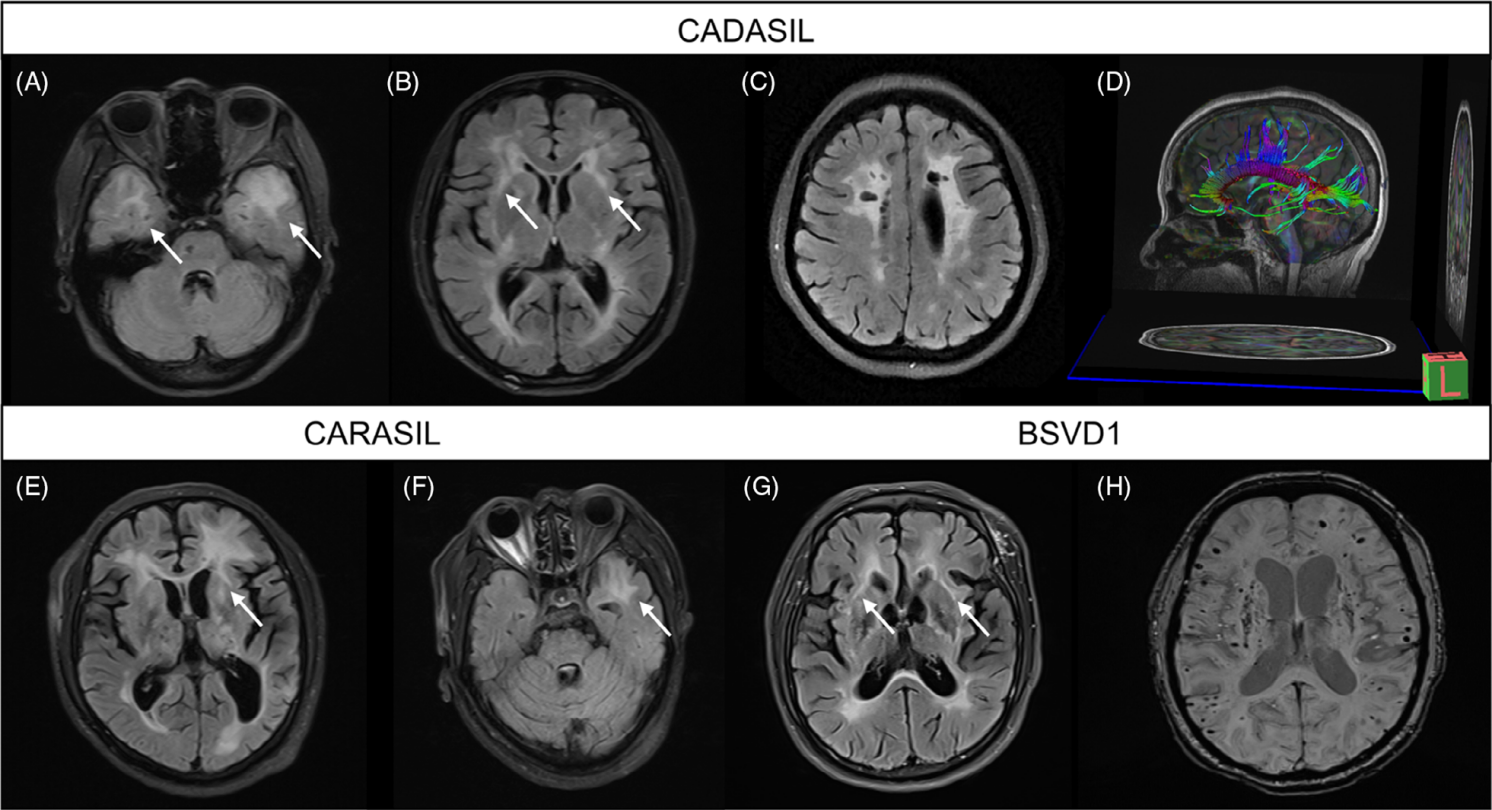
Representative MRI findings of patients with inherited vascular leukoencephalopathies. (A–D) Typical MRI findings of CADASIL patients. Axial FLAIR images show white matter lesions in the anterior temporal lobes (A), external capsules, and periventricular area (B), as well as multiple infarcts in the frontoparietal lobes (C). Sagittal DTI image shows obvious sparse and interrupted fibers in the corpus callosum bundle (D). (E and F) Typical MRI findings of CARASIL patients. Axial FLAIR images show asymmetrical white matter hyperintensities in the frontal lobe, basal ganglia, thalamus, external capsule, paraventricular area (E), as well as the left anterior temporal lobe (F). (G and H) Typical MRI findings of BSVD1 patients. Axial FLAIR image shows white matter hyperintensities in the periventricular area, internal and external capsules (G). Axial SWI image shows diffuse microbleeds in the frontoparietal lobes and basal ganglia (H). CADASIL, cerebral autosomal dominant arteriopathy with subcortical infarcts and leukoencephalopathy; FLAIR, fluid‐attenuated inversion recovery; DTI, diffusion tensor imaging; CARASIL, cerebral autosomal recessive arteriopathy with subcortical infarcts and leukoencephalopathy; BSVD1, brain small vessel disease with or without ocular anomalies; SWI, susceptibility weighted imaging.
